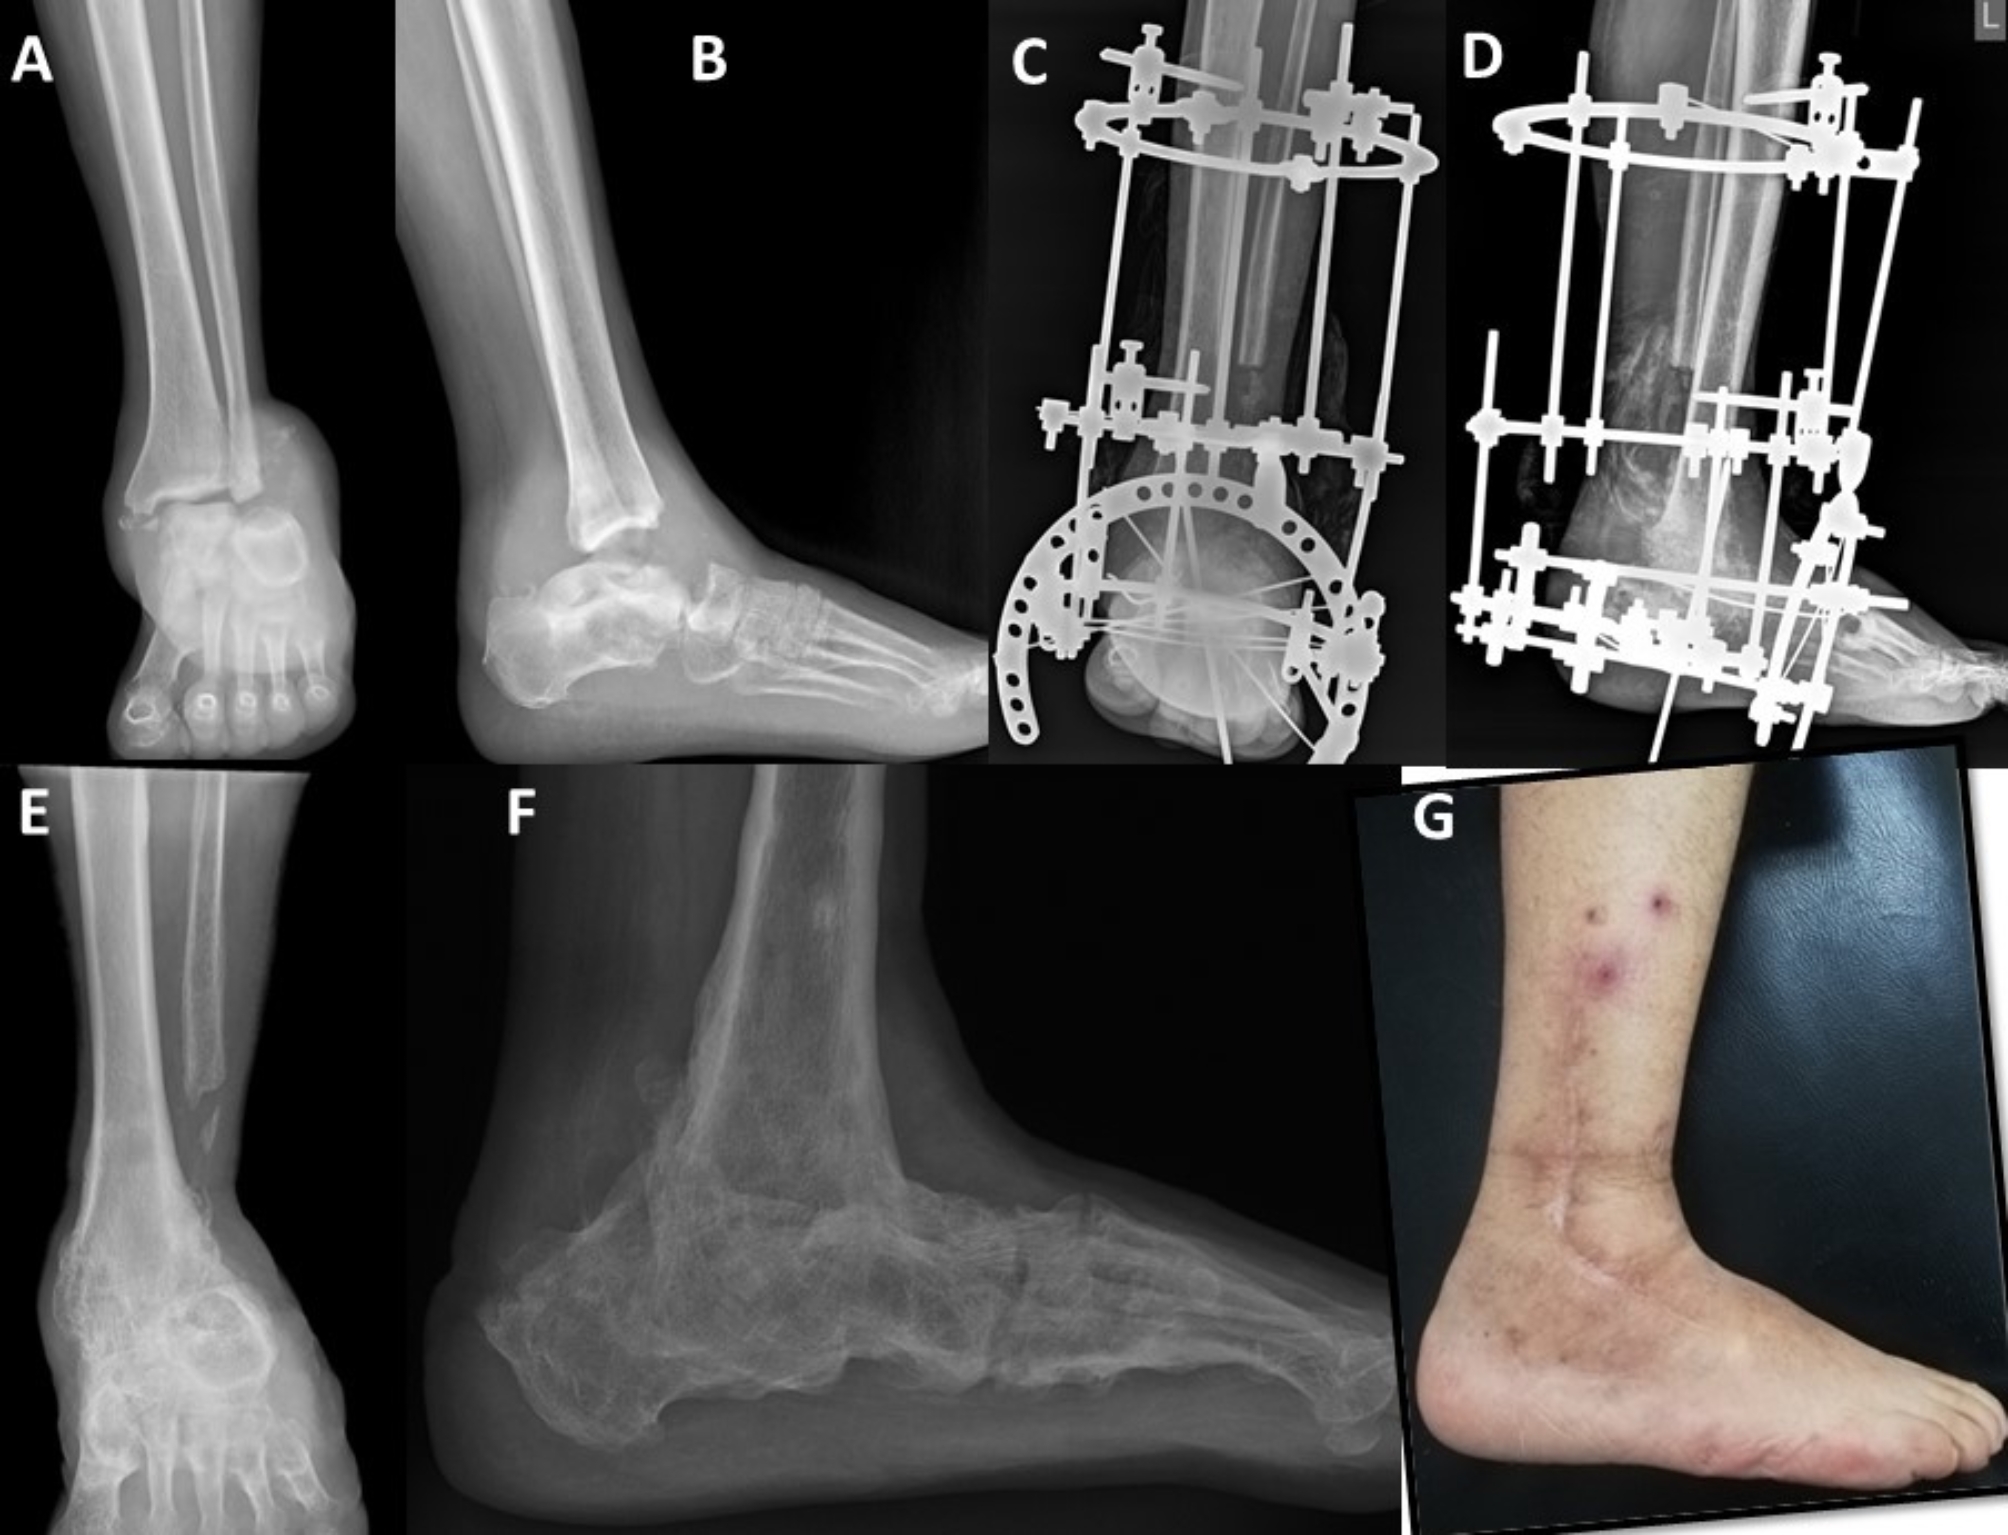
Male patient, 61 years old, diagnosed with CN of Brodsky type 3 A; he had an ulcer on the hindfoot. A and B, preoperative anteroposterior and lateral plain radiographs showing erosion of the talus and distal fibula. C and D Hindfoot fusion by Ilizarov external fixator was performed through a transfibular approach. A talectomy was performed, and the osteotomized distal fibula was used as a graft at the fusion site. E and F, radiological follow up after 30 months showing the solid bony union of the tibial and calcaneal articular surfaces. H, a clinical image showing healing of the ulcer and a plantigrade foot
